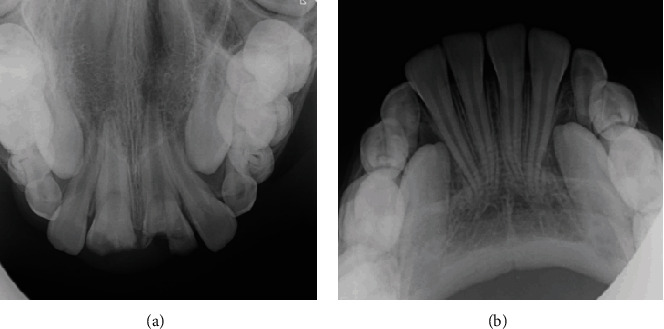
(a) Maxillary occlusal radiograph showing irregular fracture lines of maxillary teeth. (b) Mandibular occlusal radiograph showing normal dentition.
